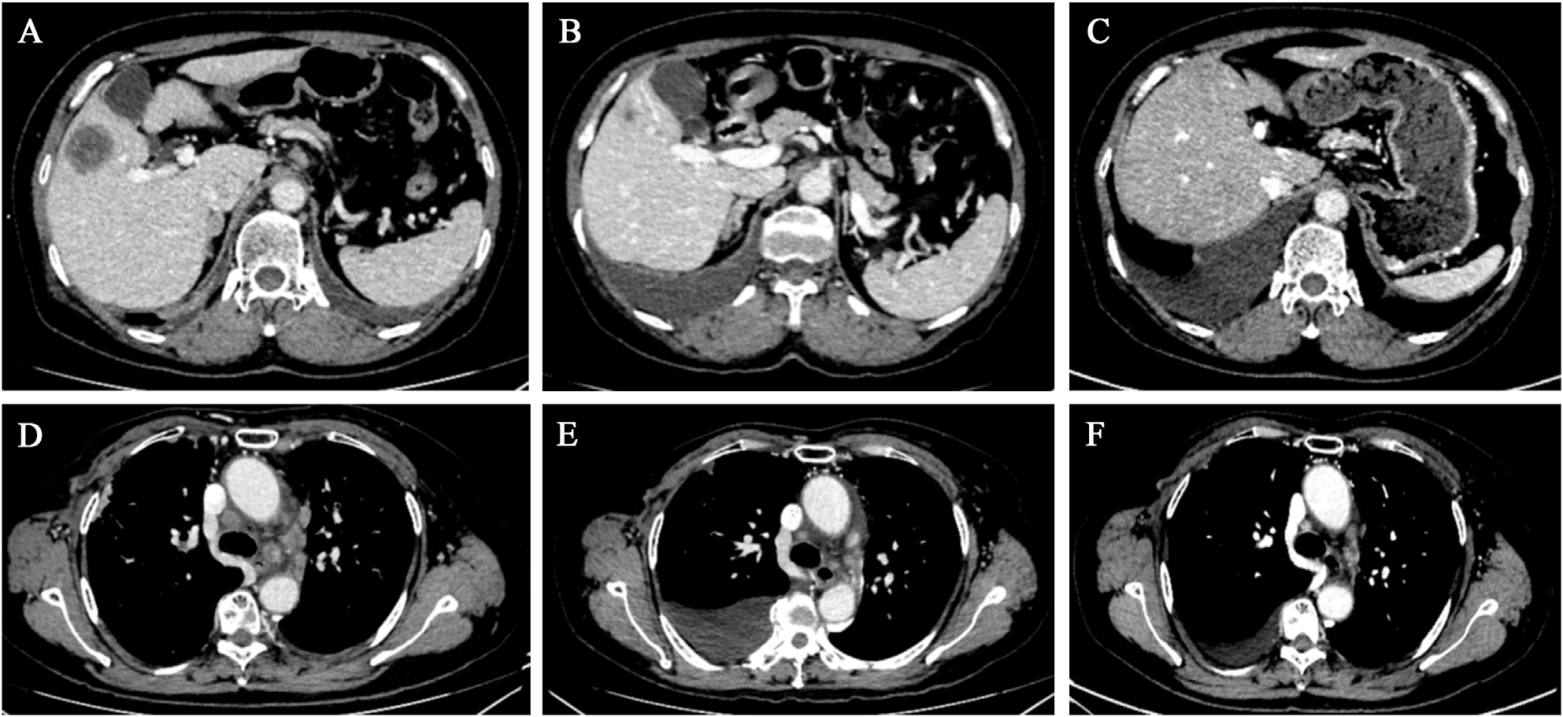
Chest and abdominal CT scans revealed a gradual reduction in the size of the hepatic metastases and mediastinal lymph nodes. (A–C) Liver cross-sectional images taken on 20 March 2023, 10 July 2023, and 14 November 2023, respectively. (D–F) Mediastinal cross-sectional images taken on 20 March 2023, 10 July 2023, and 14 November 2023, respectively.
